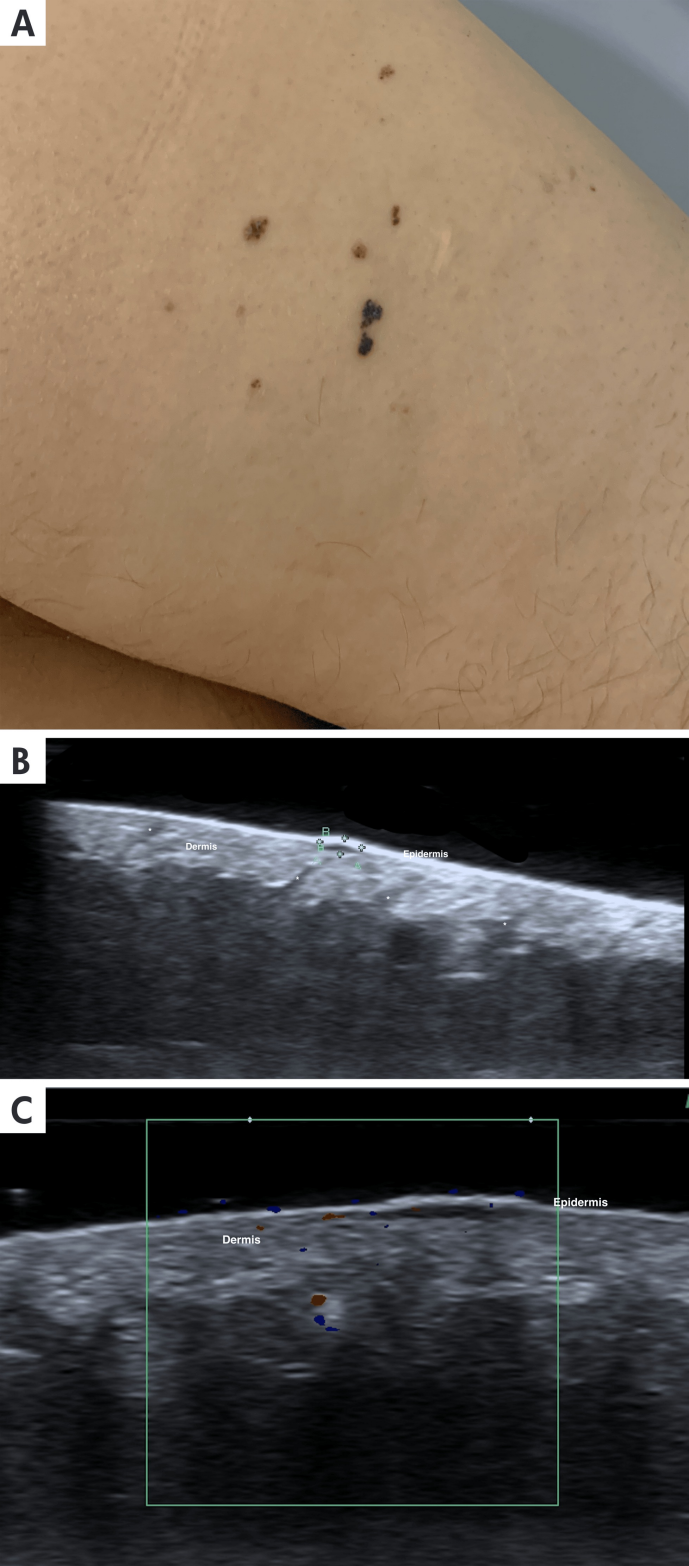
Dermatological ultrasound and clinical imaging of angiokeratoma A: A 46-year-old female patient with well-demarcated, keratotic, violaceous, and blackish papular lesions, ranging from 1 mm to 3 mm, located on the posterior aspect of the right thigh. B: The axial ultrasound view and grayscale ultrasound of the posterior aspect of the thigh show between the calipers (+) a small hypoechoic, exophytic nodule located in the dermo-epidermal junction, causing upward displacement of the epidermis. Asterisks (*) show the normal hair follicles. C. On Doppler, duplex color Doppler color ultrasound, there is a minimum increase in the vascularization on the periphery of the lesion.
